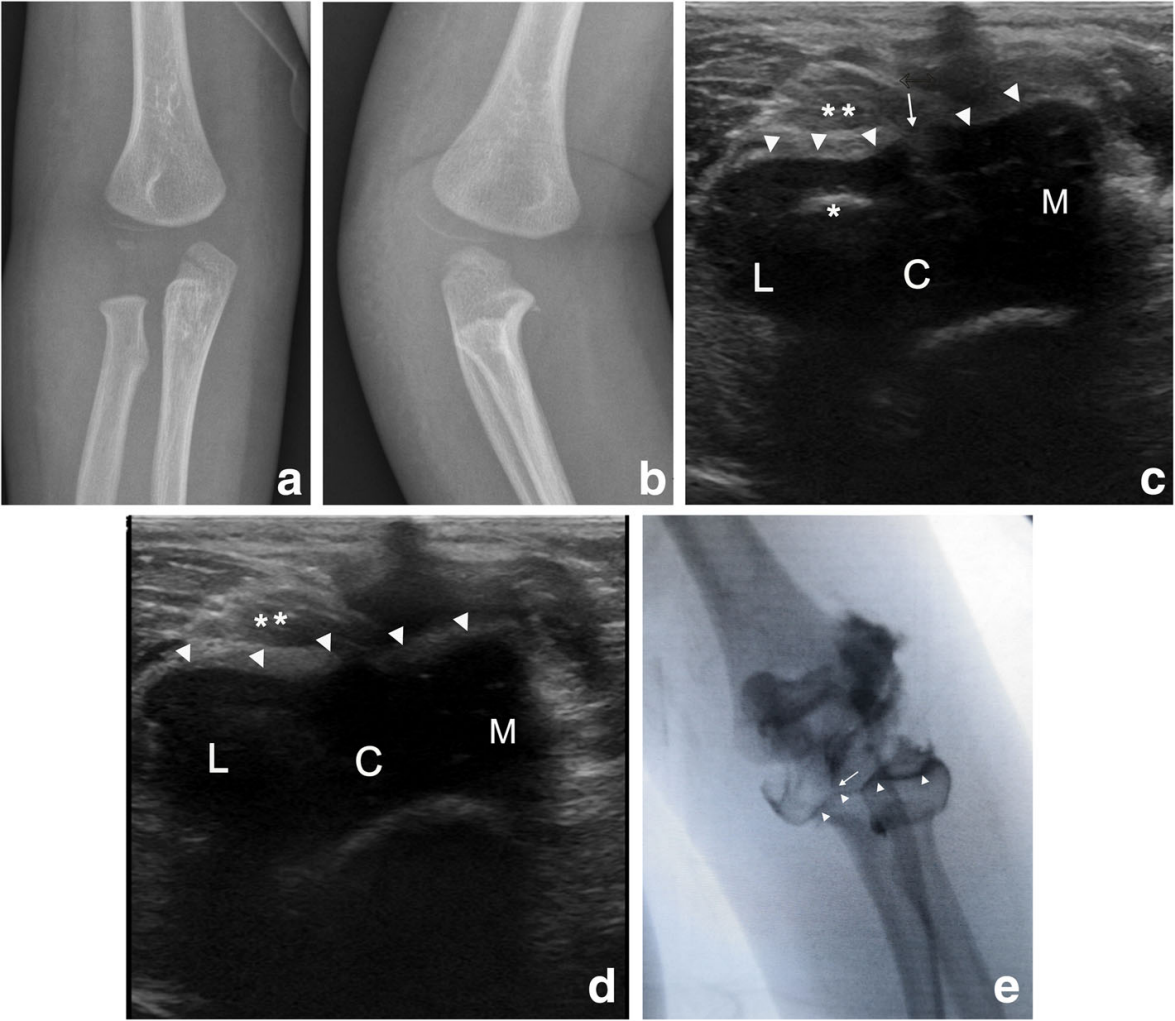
Patient case 24. a The anteroposterior radiographs showed a displacement of 2.5 mm. b The internal oblique radiographs showed a displacement of 5 mm. c The transverse ultrasound image showed the disrupted cartilage hinge on lays when the center of the ossification region in the capitellum epiphysis was found. d The transverse ultrasound image showed the intact cartilage hinge before reaching the articular surface and the disrupted cartilage hinge (arrow). e Radiographs in the anteroposterior views of an arthrogram. The dye was tracked along the fracture line and stopped before reaching the articular surface (arrow), thus indicating an intact cartilaginous hinge. Arrowhead, cartilage hinge; asterisk, epiphyseal core of ossification of the capitellum; double asterisks, hemorrhage; M, medial; L, lateral; C, cartilage of the epiphysis
